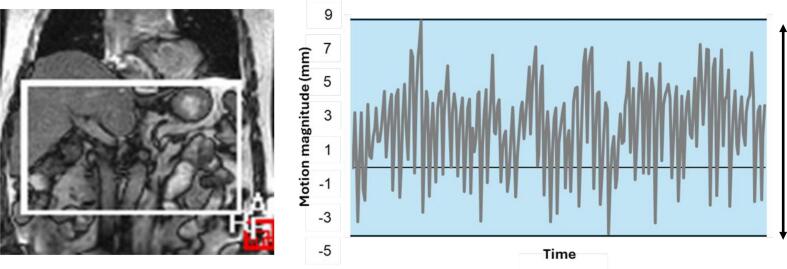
Left, sample registration clipbox on a single coronal cine-MRI for a patient in the registration software MatPel. Right, sample of raw motion data plotted as pseudo-motion trace, the arrows and shaded area represent the maximum range of motion used in this study.
